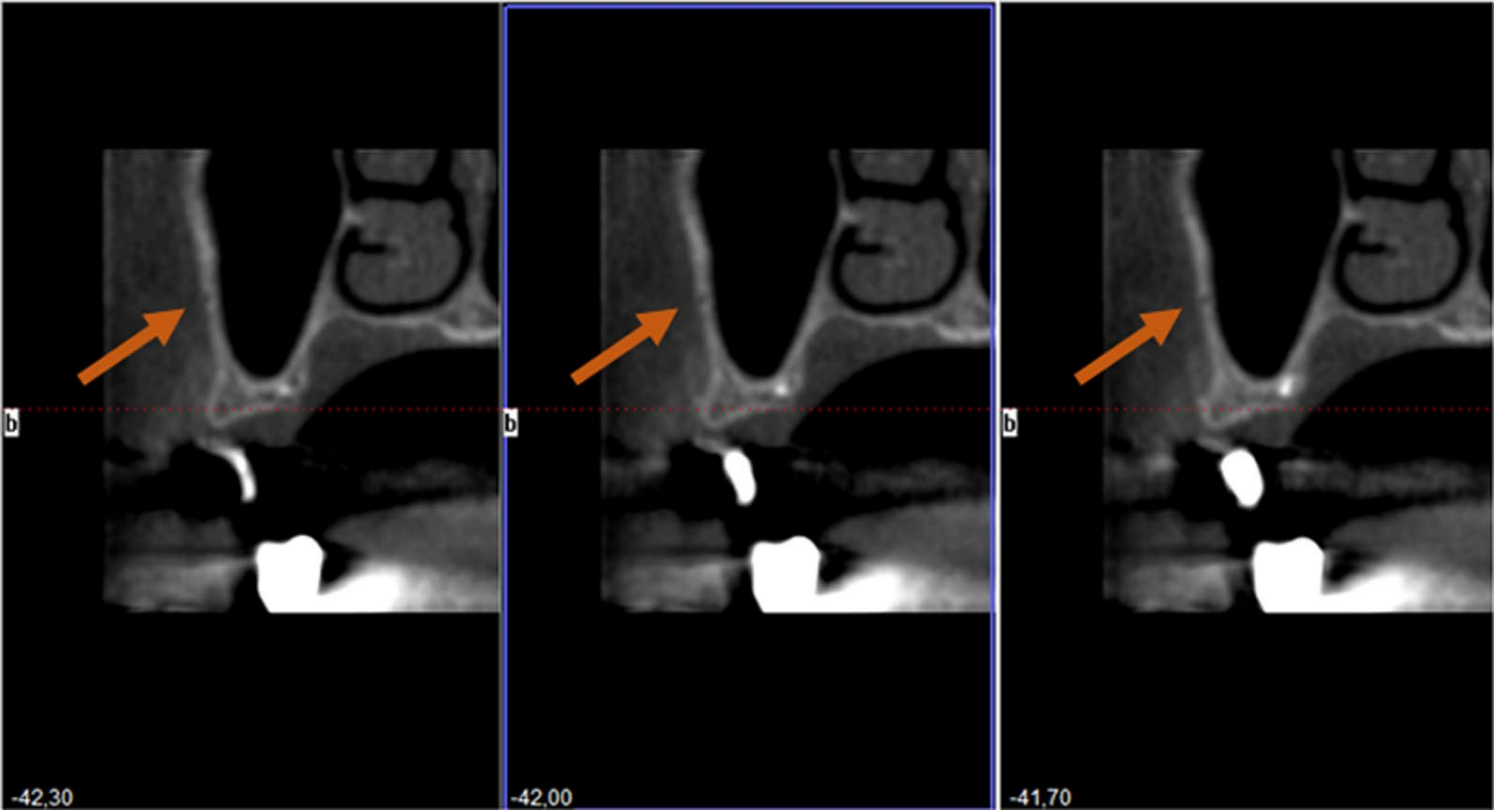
CBCT images with AAA canal of the right maxillary sinus, coronal slices
